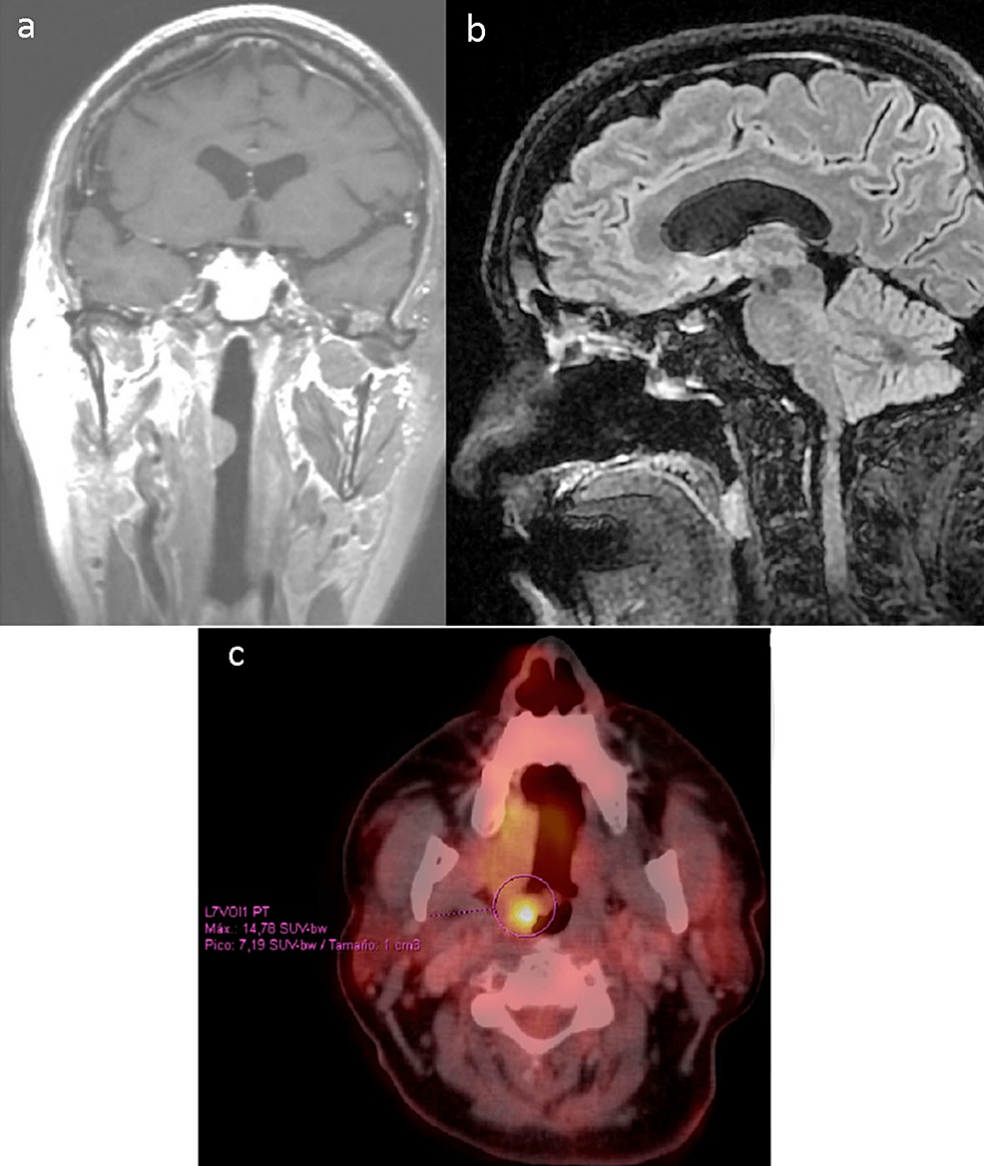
MRI images showing a right oropharyngeal lesion partially behind the soft palate (frontal a, sagittal b). PET-CT with isolated metabolic activity (axial c). PET: Positron emission tomography.
